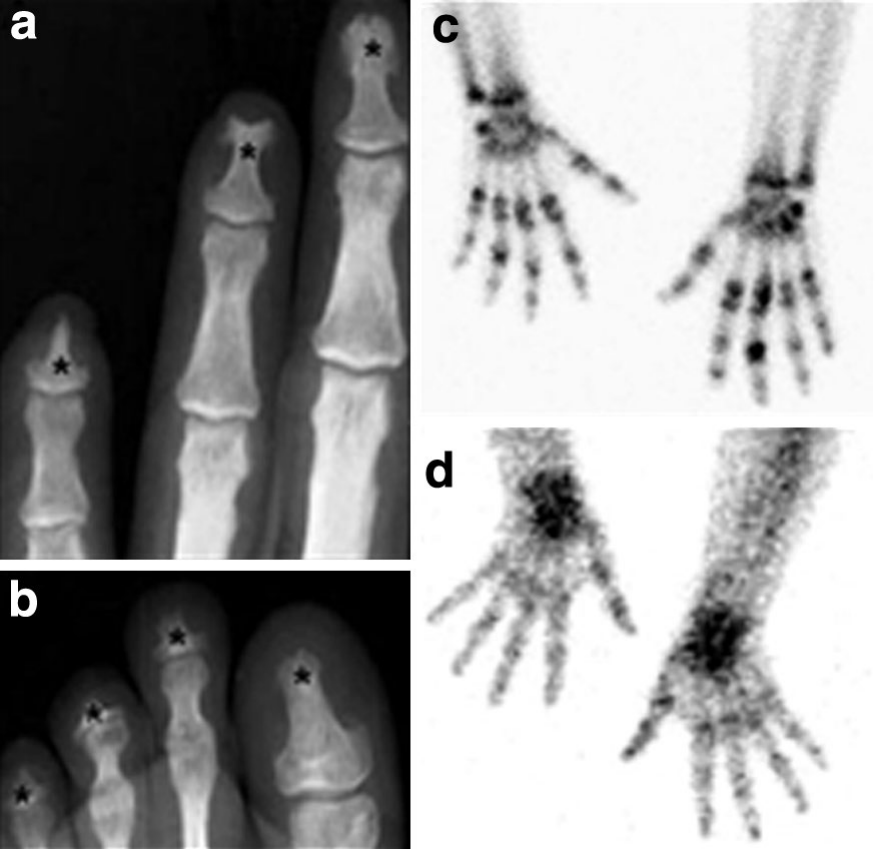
Newly diagnosed lepromatous patients exhibits bone and cartilage lesions. a, b Radiography of left hand and foot. *Asterisks* indicate the distal phalanx erosion, typical hallmark of leprosy. c Third-phase bone scintigraphy image shows increased bone uptake of ^99m^Tc-MDP in both hands. Hyper-fixation occurred in bones and joints of phalanges, metacarpus and wrists. Important joint alterations were evidenced in all patients. d The early phase scintigraphy shows localised radiotracer activity on the wrists
